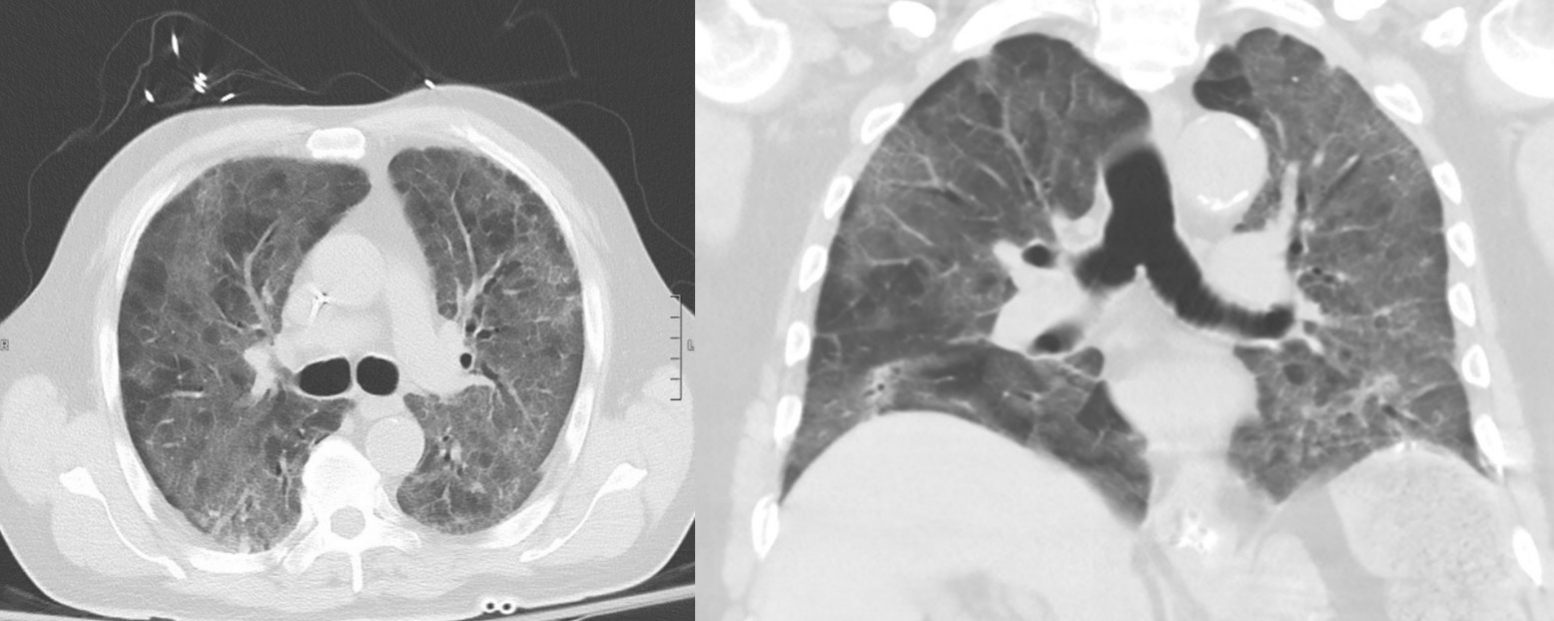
Representative slices of CT chest obtained on hospital Day 12 showed more diffuse ground‐glass opacities with peripheral clearing and reticulations consistent with organization and early fibrotic changes
